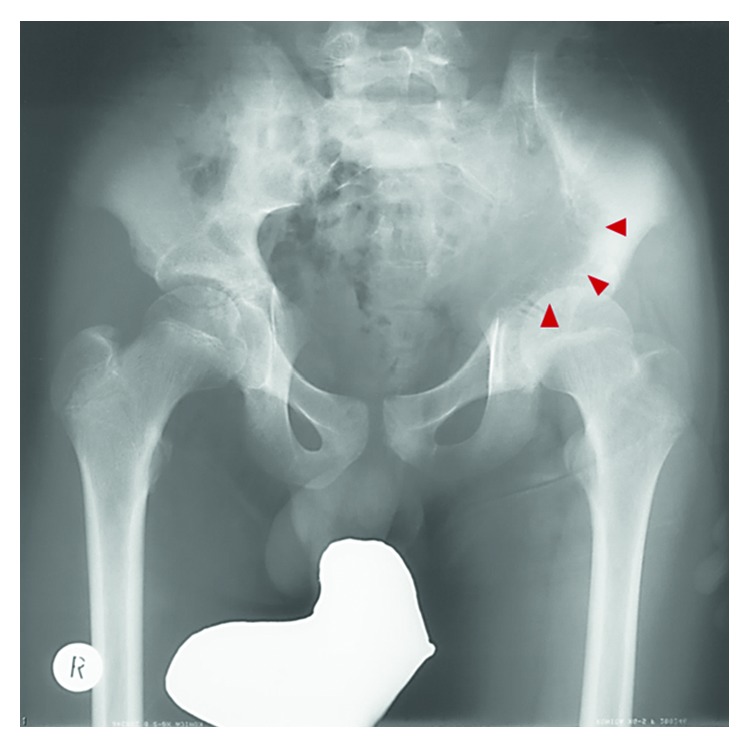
Plain radiograph showing osteolytic changes at the medial side of the left iliac bone.
